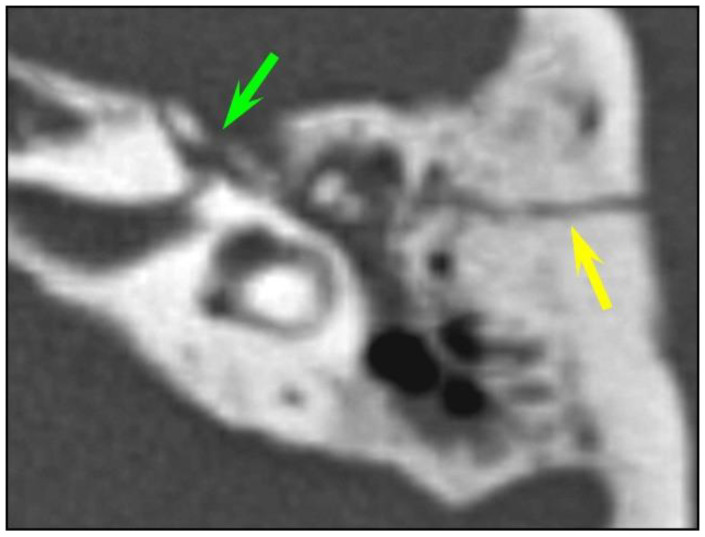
Facial canal injuries. Fracture of the left temporal bone in a 25-year-old man with peripheral facial palsy after a fall. CT of the left temporal bone: longitudinal fracture (yellow arrow) with associated bony fragments in the geniculate ganglion fossa (green arrow) consistent with a geniculate facial canal injury.
